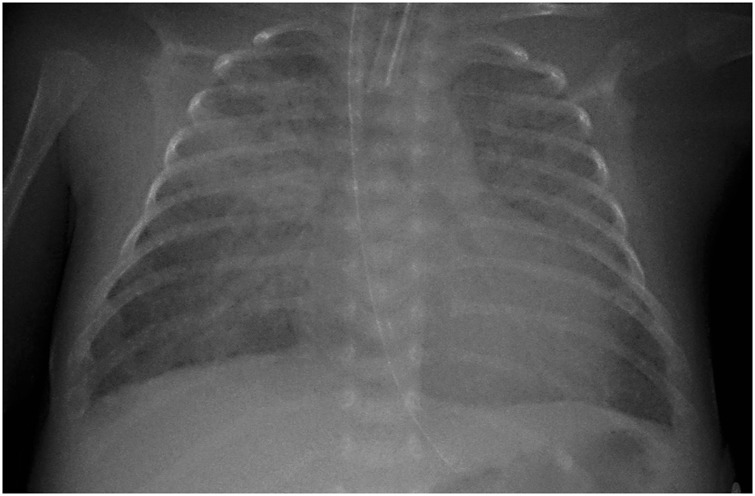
Chest radiograph in a ventilated preterm infant with a large PDA. Note the large heart shadow and the increased lung markings representing pulmonary overcirculation
